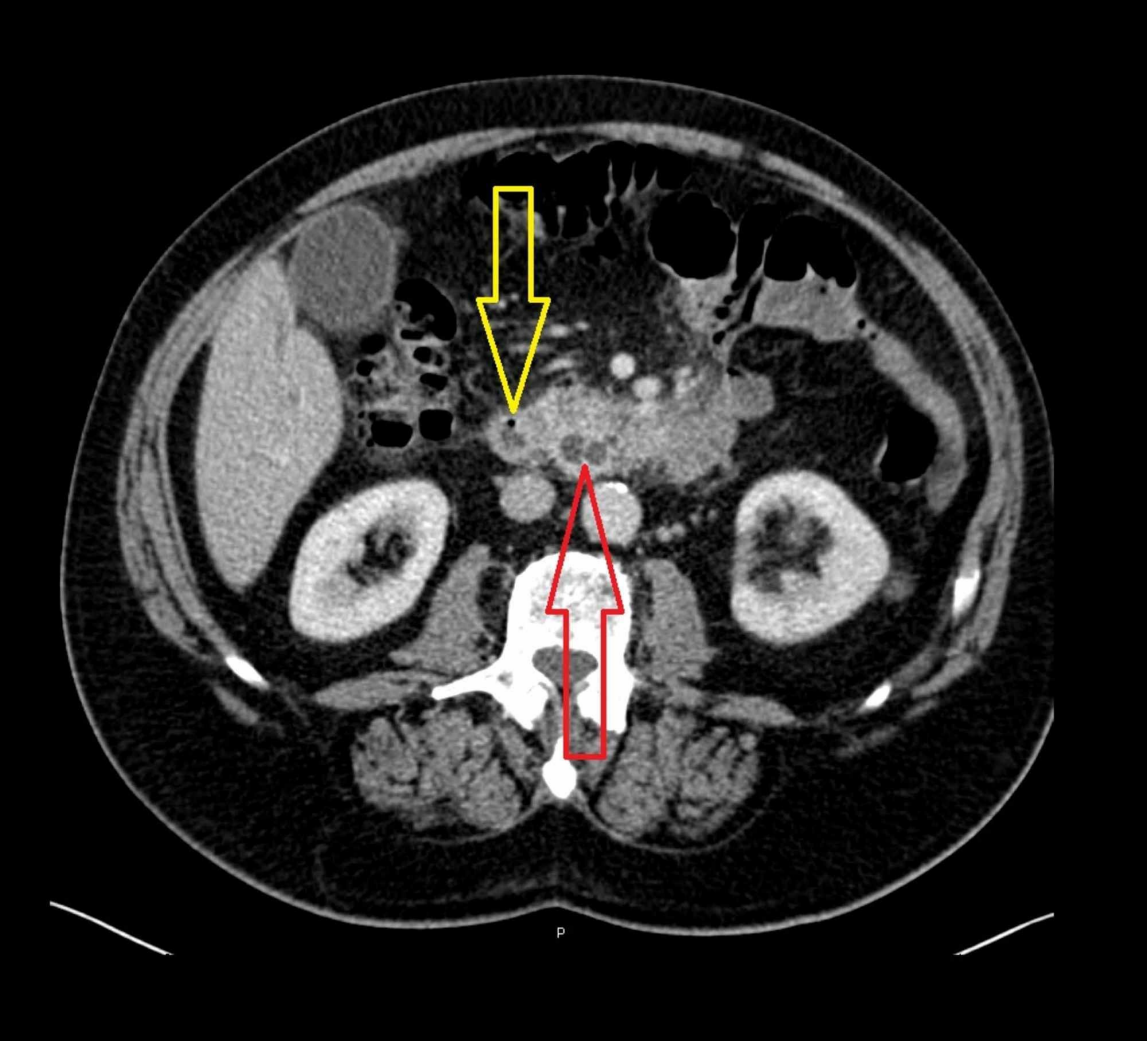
Abdominal CT scan. The red arrow indicates the dilatation of the common bile duct (CBD) and major pancreatic duct (MPD) caused by the endoluminal duodenal mass (yellow arrow). CBD: common bile duct. MPD: major pancreatic duct
